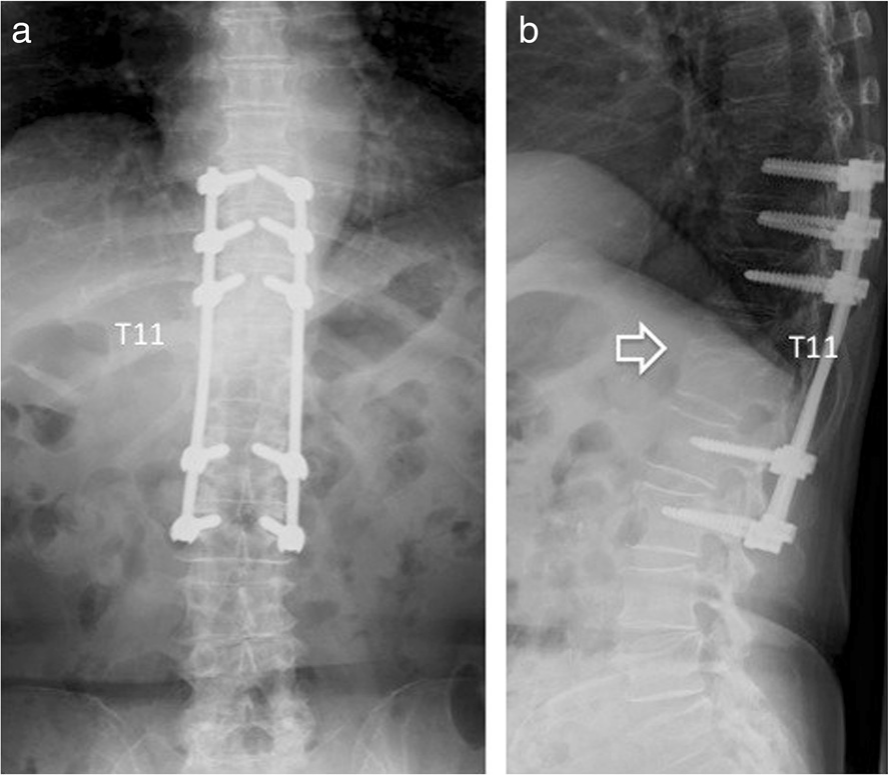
Plain radiograph at 18 months after operation. Complete union between the T11 and T12 vertebral bodies is seen. Open arrow shows bone bridge between T11 and T12 vertebra. (a) Anteroposterior radiograph, (b) Lateral radiograph.
